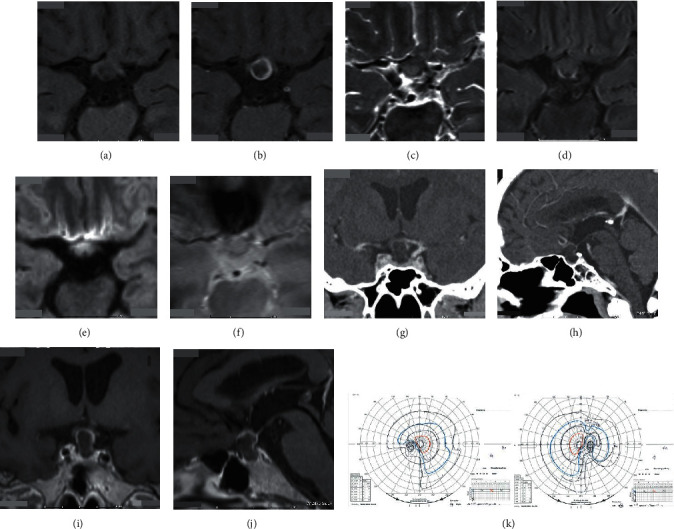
Precontrast T1-weighted axial image showing a suprasellar tumor compressing the optic pathway (a). Gadolinium-enhanced axial image showing an enhanced rim of the tumor (b). The contents of the tumor are of low signal intensity on the T1-weighted image (a) and Gadolinium-enhanced image (b), slightly high on T2-weighted image (c), iso on fluid-attenuated inversion recovery image (d), and relatively high on diffusion-weighted image (e) and T2∗-weighted image (f). Contrast-enhanced computed tomography showing a cystic lesion (g) from the sella turcia to the suprasellar space (h). Calcified components are not found in it. Postcontrast magnetic resonance imaging shows the enhanced cystic wall displacing the optic chiasm upward (i). This cystic lesion occupies the suprasellar region but not the third ventricle (h), (j). Goldmann perimeter reveals irregular bitemporal hemianopsia (k). Constriction of the visual field is more predominant in the right eye than in the left eye (k).
